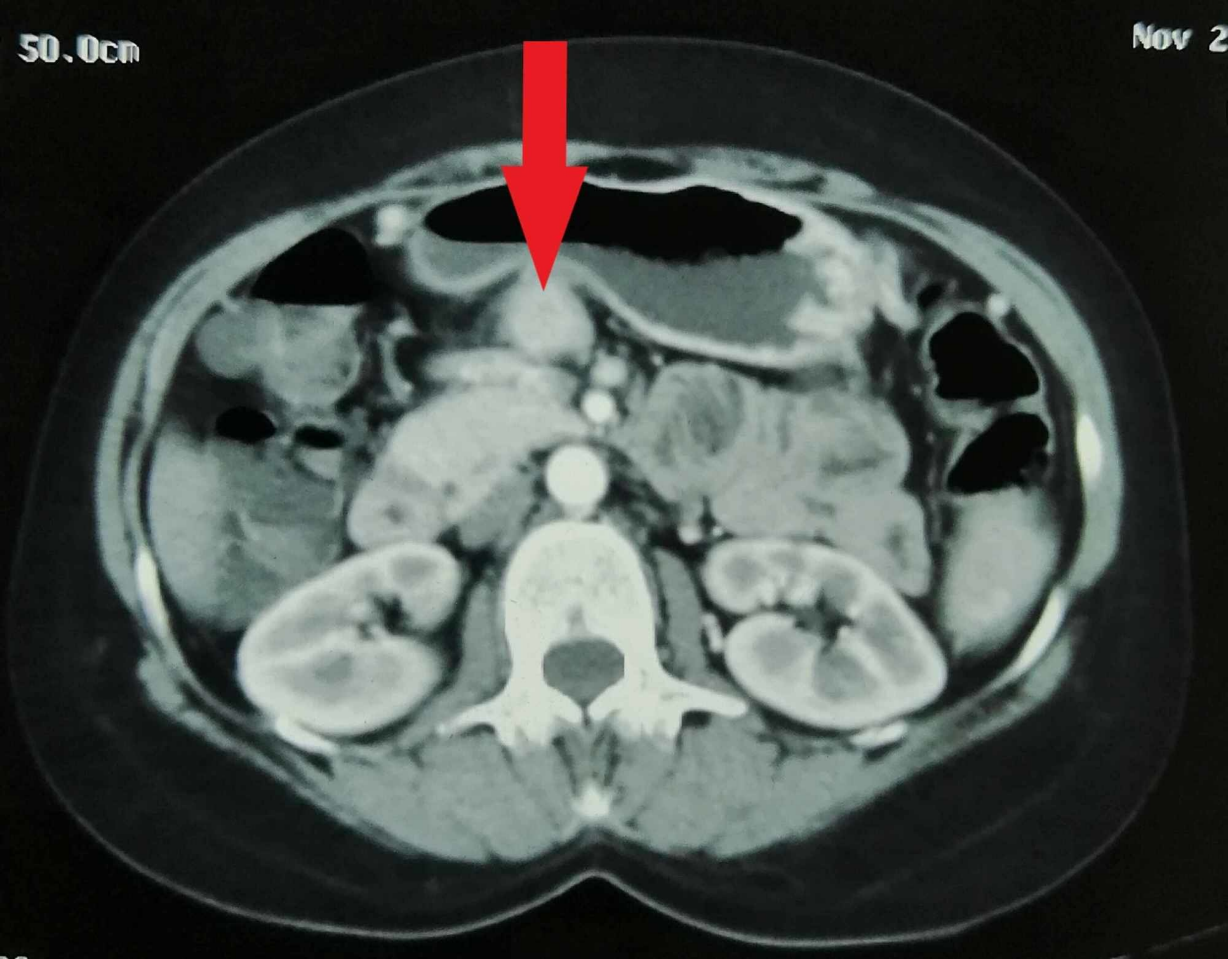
Axial view of abdominal CECT showing a hyperdense, ill-defined mass arising from the pancreatic body CECT, contrast-enhanced computed tomography
